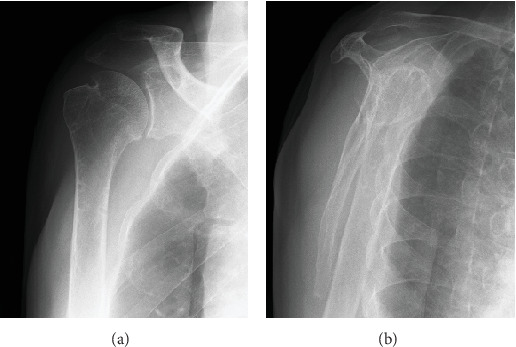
Plain postoperative radiographic images. Plain postoperative radiographic images taken at 24 months postoperatively. (a) AP view, (b) Y view.
